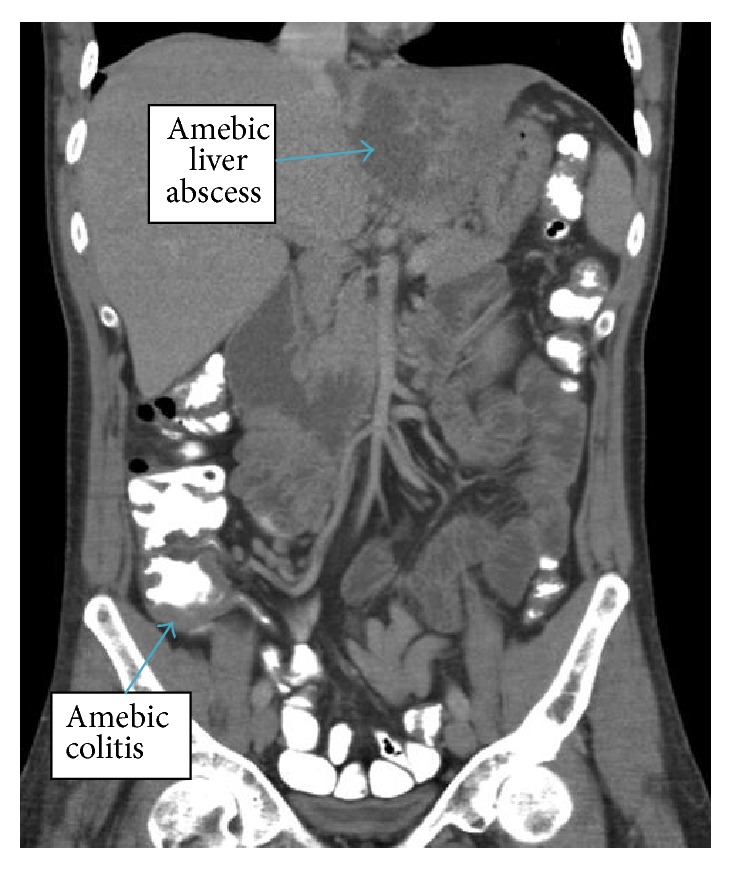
CT abdomen showing 8.5 × 9.6 × 6.8 cm liver mass and cecal wall thickening suggestive of colitis.
